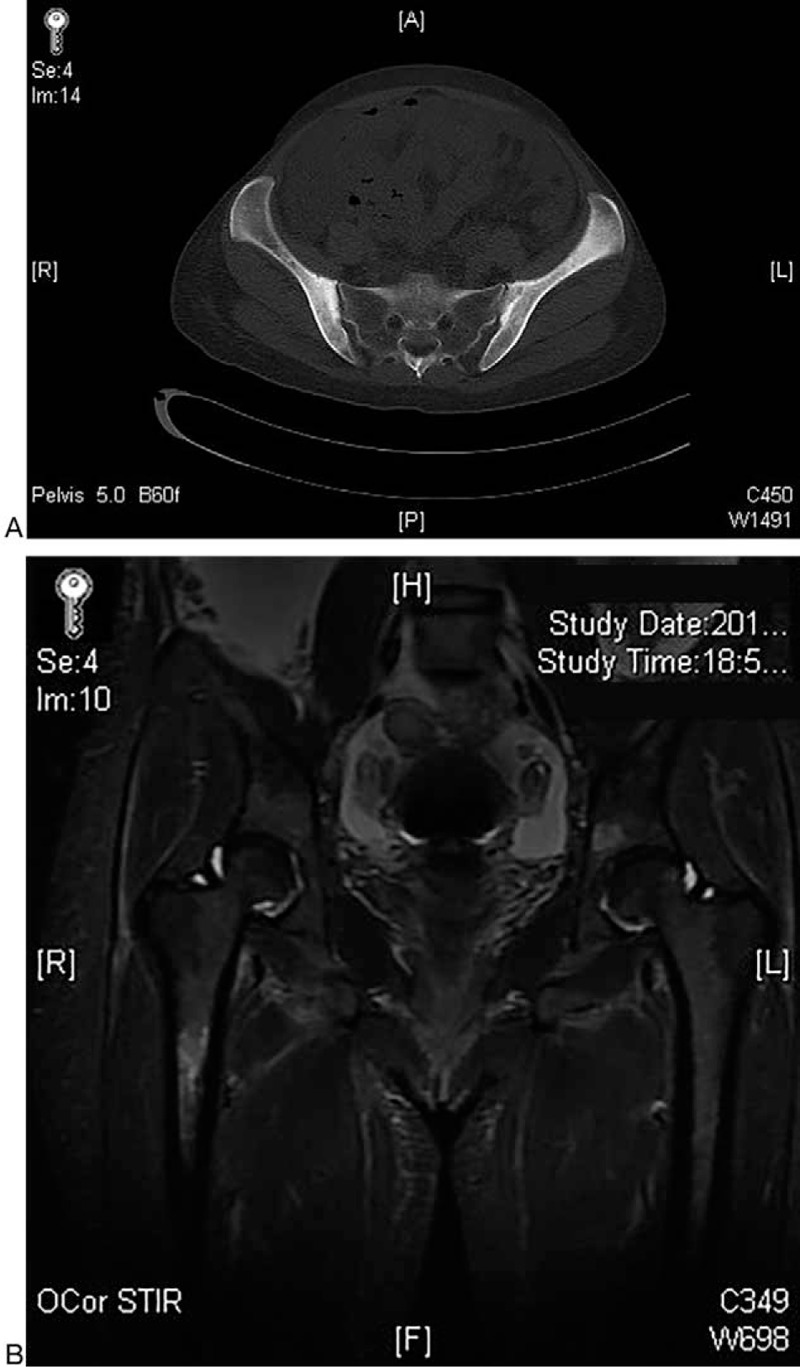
Imaging studies. (A) The ilia show mildly expansile change with thickened and blurred cortex on contrast-enhanced computed tomography (CT) scan, which indicates ossification and bilateral sacroiliitis, greater on the left. (B) Edema of bone marrow was observed in the right proximal femur, which manifested as increased signal intensity in the short tau inversion recovery (STIR) images.
